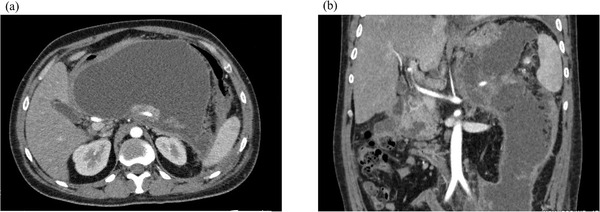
Abdominal contrast‐enhanced computed tomography (pre‐treatment). (a) Massive acute necrotic collection is evident in the upper abdomen. (b) The acute necrotic collection extends continuously from the upper abdomen into the pelvic cavity
